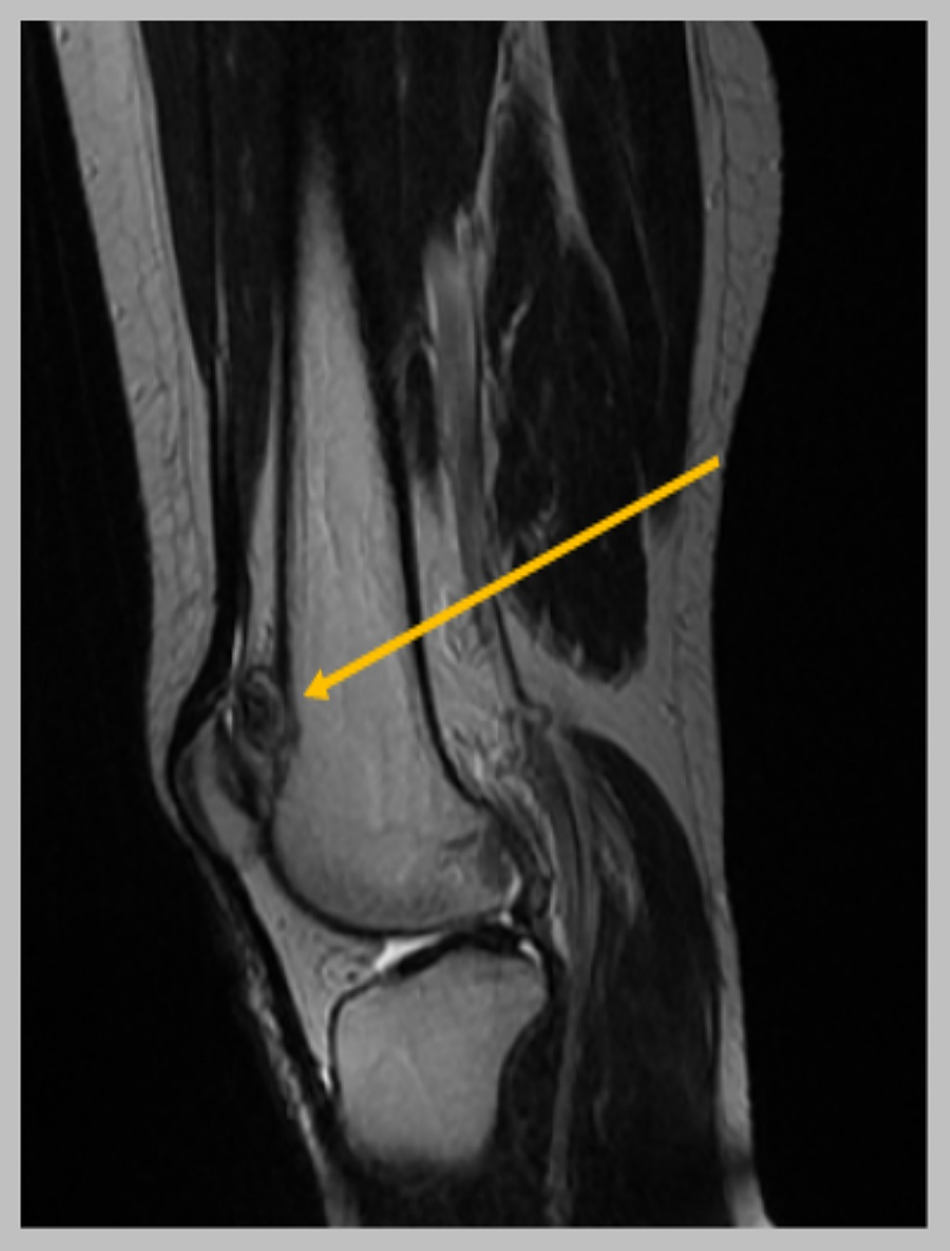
MRI sagittal plane showing the lesion (yellow arrow). It can be seen that the lesion is arising from the femoral bone, on the superior edge of the patellofemoral joint.
